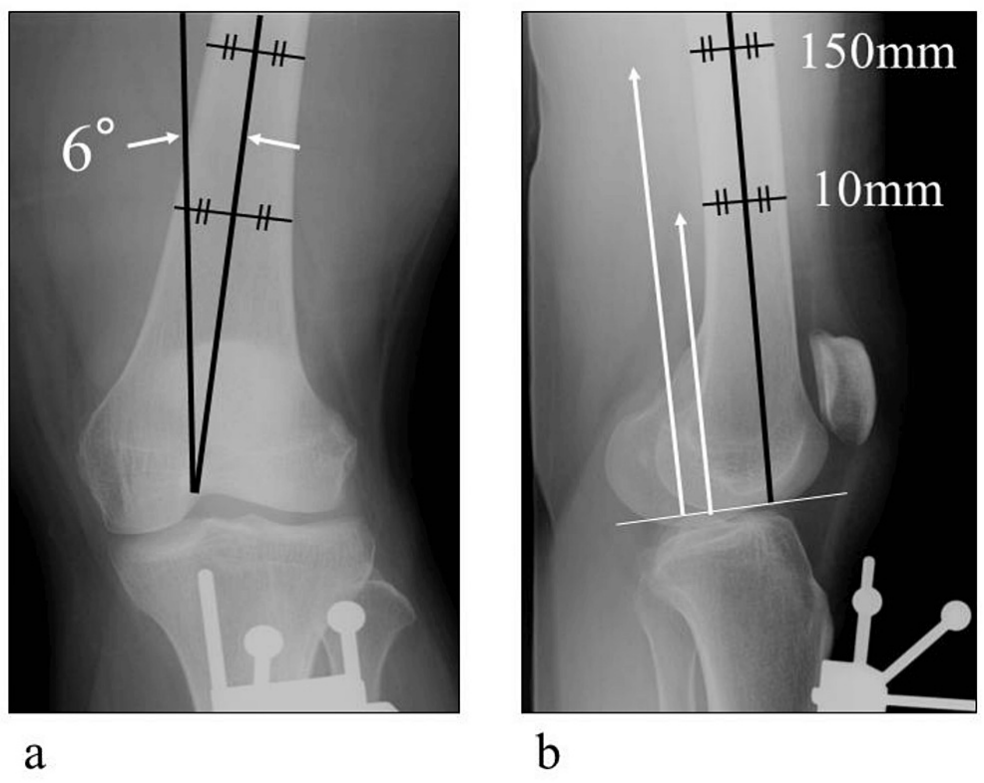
Definition of the radiographical axis. (a) The femoral mechanical axis was defined as the angle of 6° valgus to the femoral anatomical axis. (b) The femoral anatomical axis was defined as the line at the midpoint of the femoral width at 100 mm and 150 mm proximal to the distal joint line.
